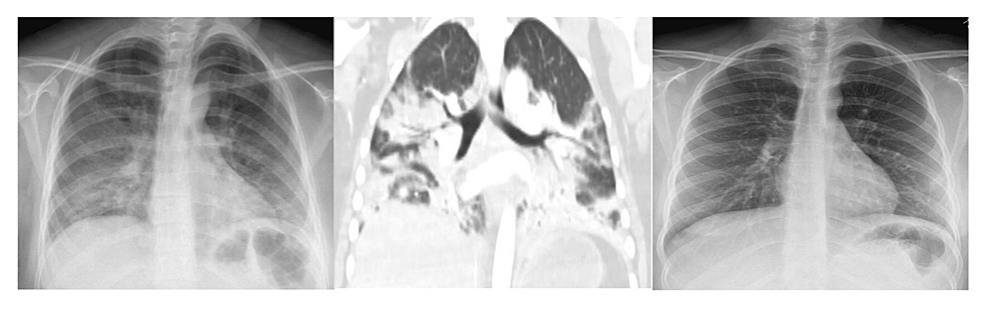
Left: CXR on presentation. Middle: Chest CT showing bilateral patchy consolidation and GGO on presentation. Right: Follow-up CXR after a month. CXR: chest X-ray; CT: computed tomography; GGOs: ground-glass opacity
